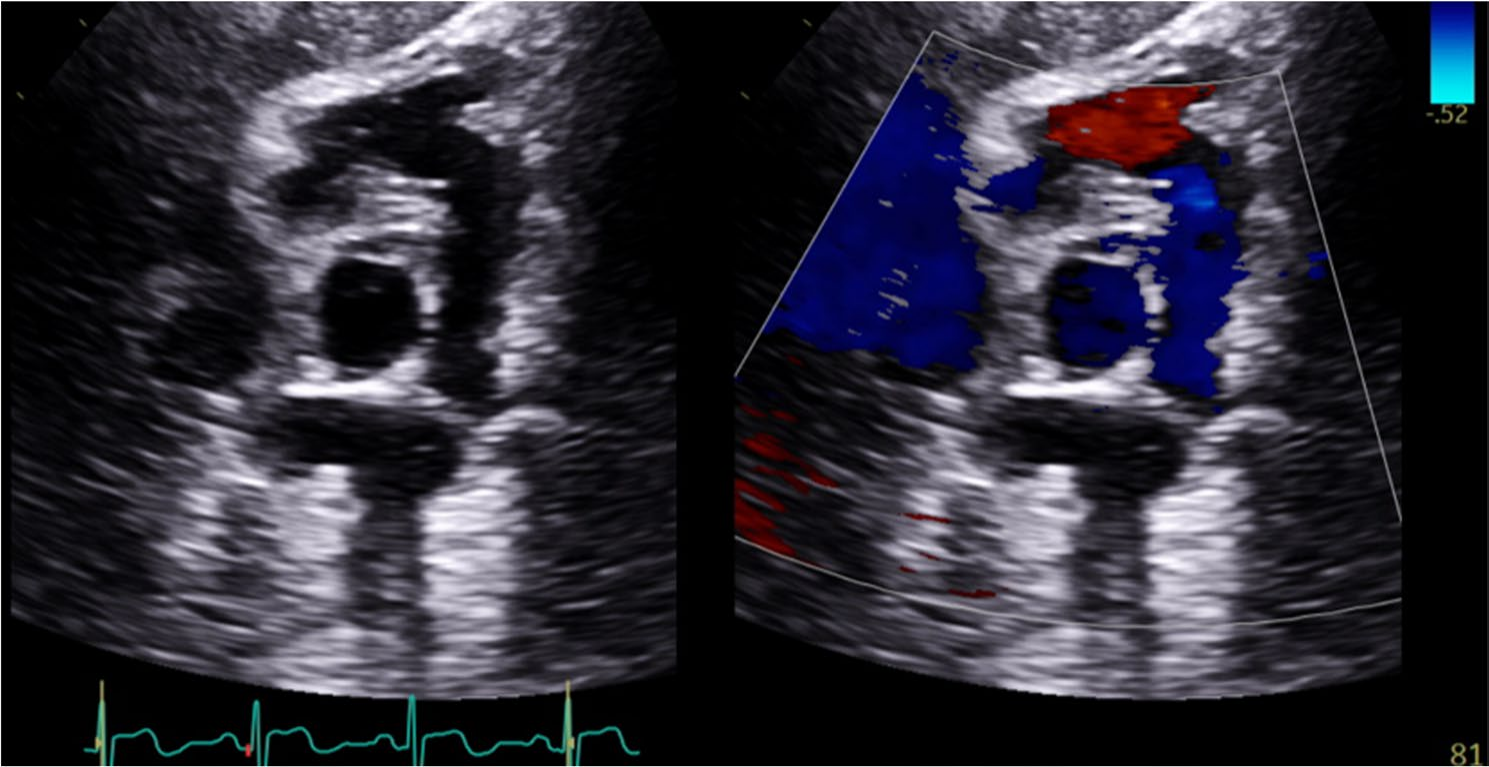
Post-operative TTE in modified parasternal short-axis with simultaneous 2D and color doppler of the PV demonstrating PV/RVOT repair. No PV regurgitation or stenosis was demonstrated in this study
